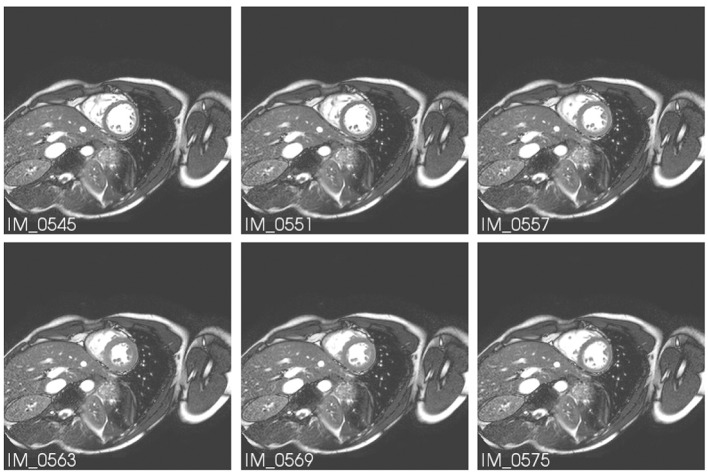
Six MR images at different phases of cardiac cycle (it is possible to observe the movement of the myocardium). Extracted from Koch et al. (2011).
